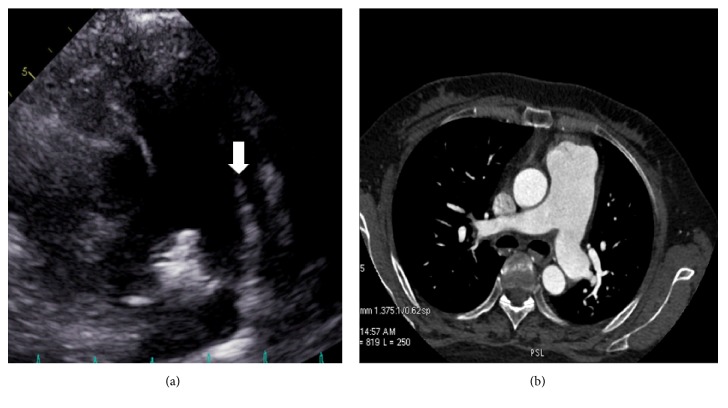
(a) Aortic short-axis and pulmonary artery long-axis views. A linear artifact is clearly seen (white arrowhead). (b) Computed tomographic pulmonary angiography shows that the main pulmonary artery and left and proximal right pulmonary arteries are clear with no artifacts.
